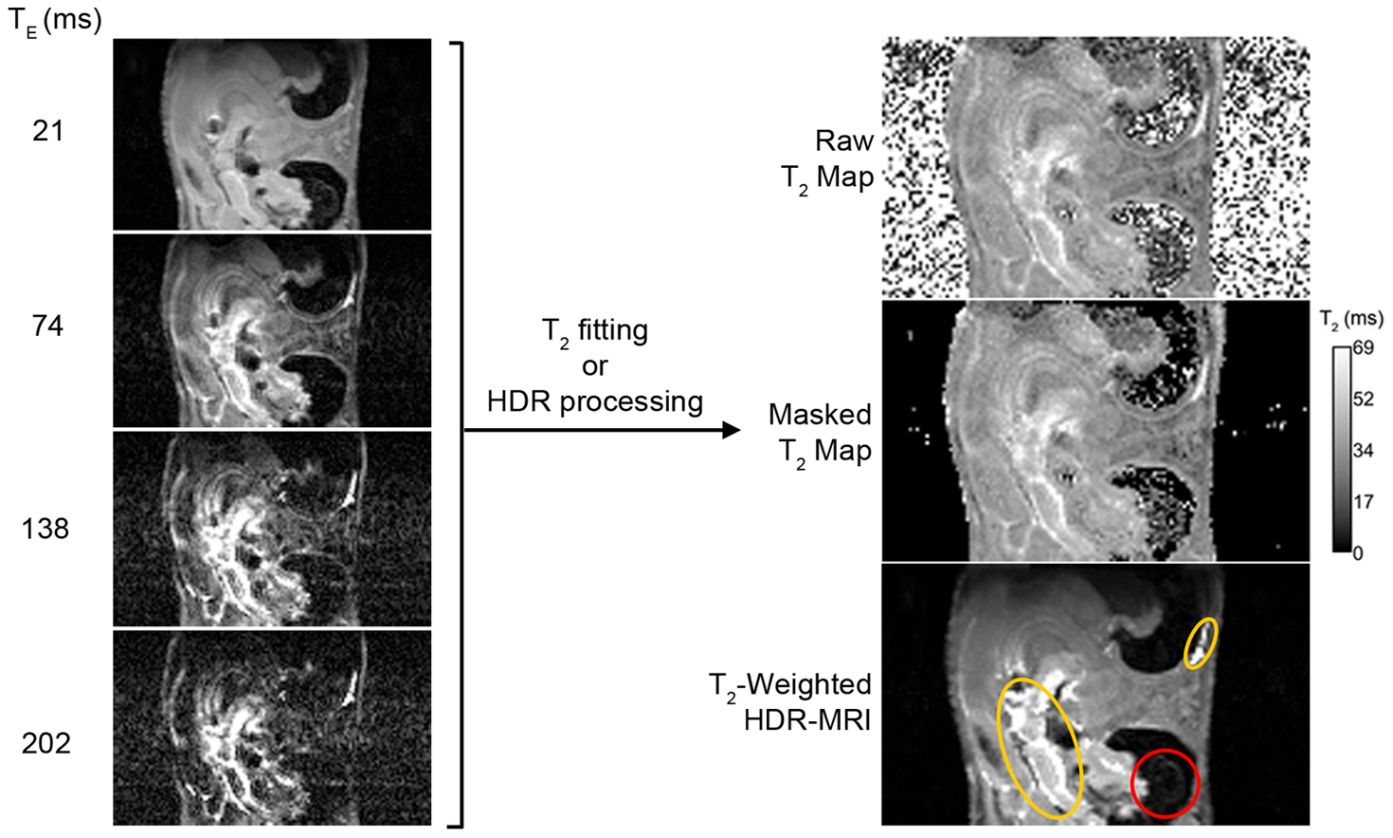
In vivo* HDR-MRI. A series of images were acquired with constant T_R_ at 5632 ms and varying T_E_ as indicated. The same four LDR images were used to generate both the T_2_ map and the HDR-MR image. Masking of the T_2_ map was done by manual thresholding. In the HDR image, red and yellow outlines highlight features that were not captured in one or more of the individual LDR images. HDR-MRI captures the same features as T_2_ mapping, but is less noisy in the low signal regions. Low signal features can be accurately depicted in HDR-MRI even when the features are only visible in a single LDR image.
